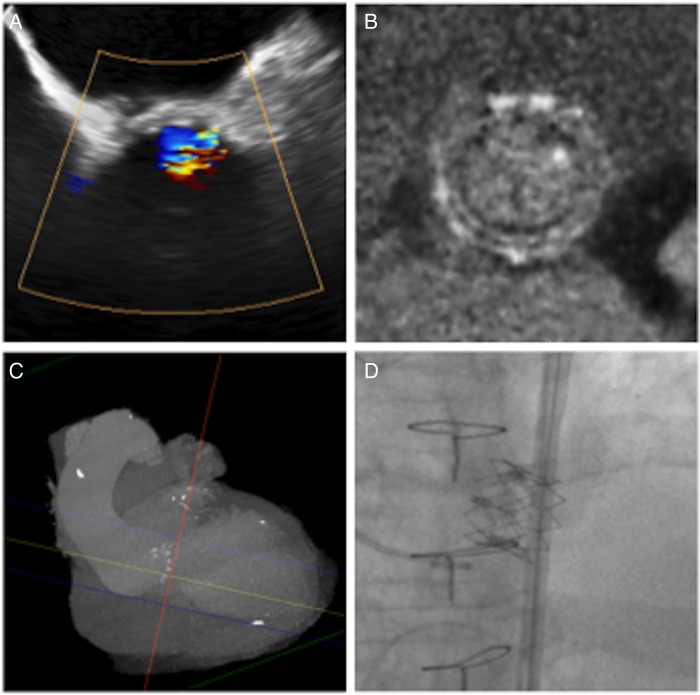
An example adjunctive CT imaging in the management of aortic valve replacement dysfunction. (A) Severe prosthesis regurgitation on transoesophageal echocardiography resulted from calcific structural valve dysfunction of the bioprosthetic leaflets in a 27 mm Aspire bioprosthesis demonstrated on CT (B). (C and D) Preprocedural planning facilitated implantation of a transcatheter aortic valve in valve.
